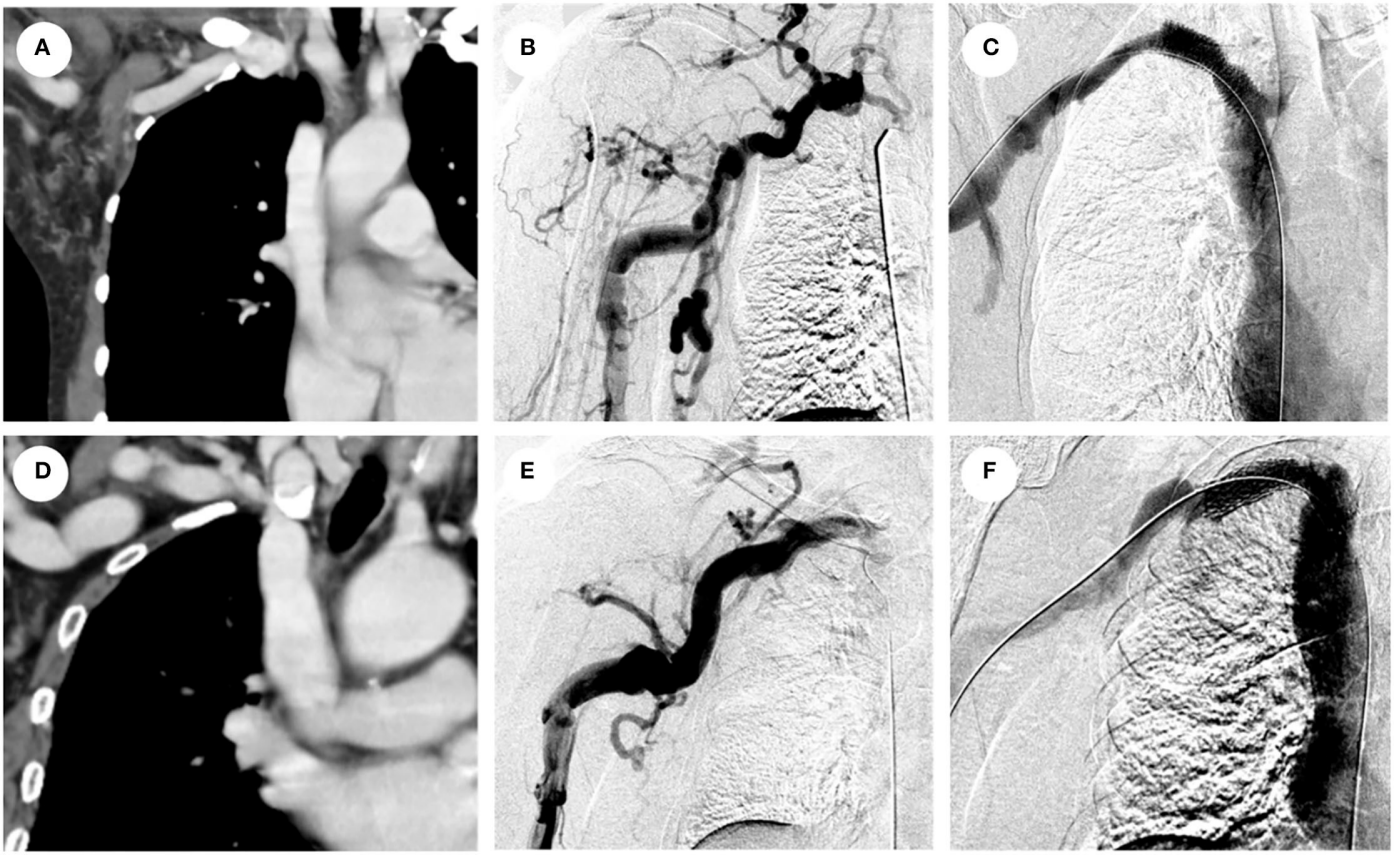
Representative images of CVO recanalization in cluster 1 and cluster 2. (A) Computed tomography of (CT) reconstruction of long segmental occlusion exceeding 2cm with blunt stump and calcification in the right brachiocephalic vein; (B) venography shows multiple collateral circulations around the occlusion; (C) venography showing unobstructed blood flow in the right brachiocephalic vein after covered stent placement; (D) CT reconstruction of stenosis <2 cm in the right subclavian vein without calcification and blunt stump; (E) venography showing stenosis without collateral circulation within 1 cm; (F) venography showing unobstructed blood flow in the right subclavian vein after covered stent placement.
